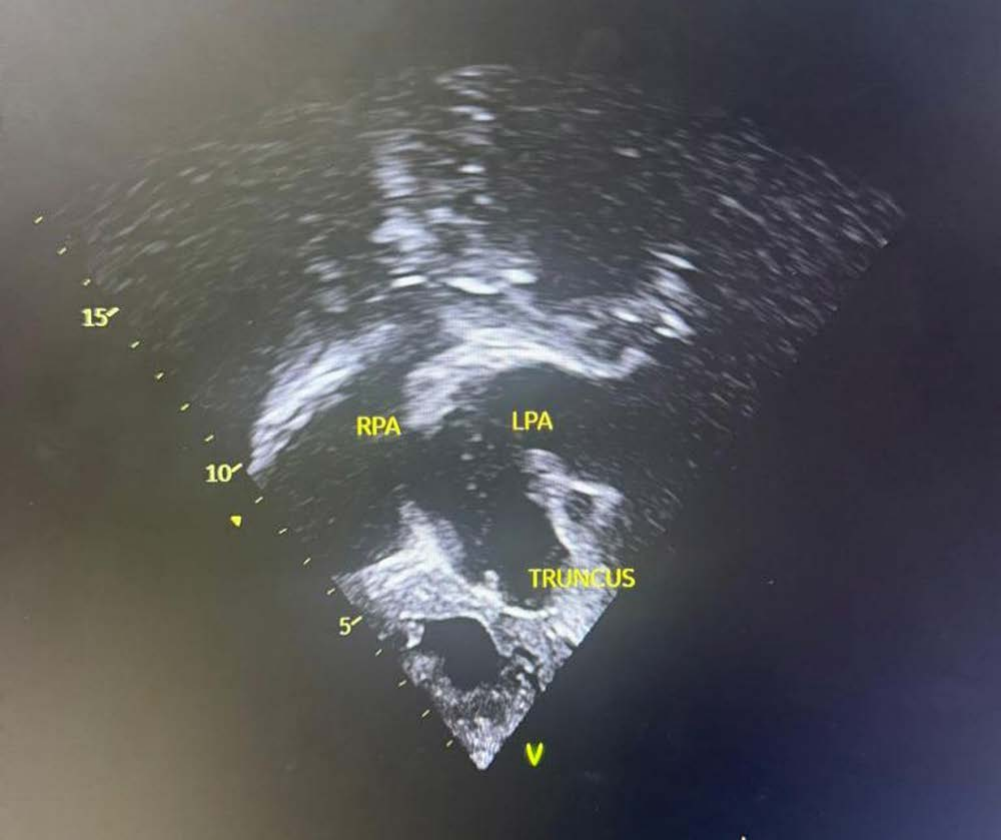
Echocardiographic image showing prominent truncus and right (RPA) and left pulmonary artery (LPA).
